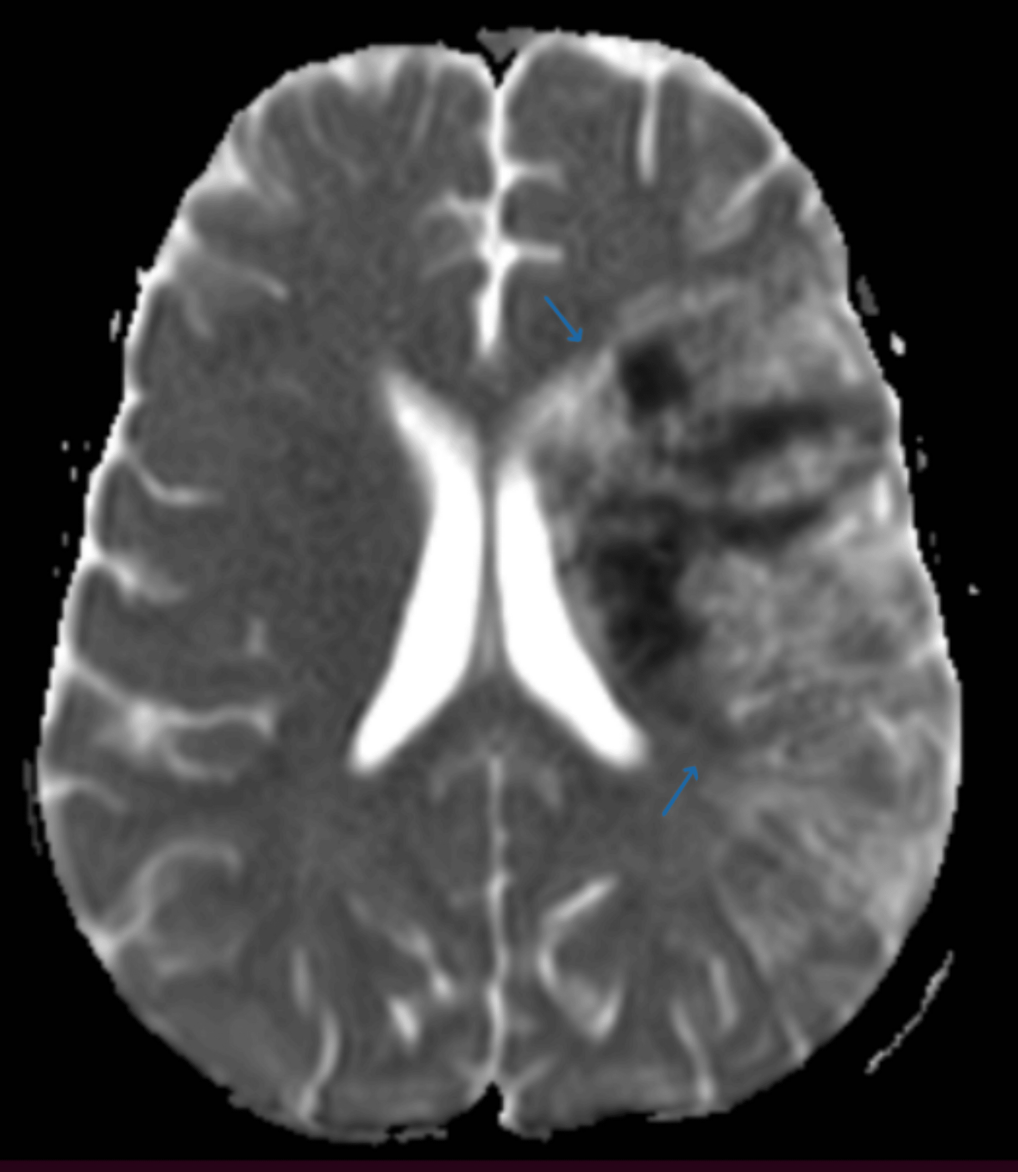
Axial T2-weighted MRI brain showing hyperintense signal in the left frontoparietal cortex and subcortical white matter, consistent with acute infarction involving the left MCA territory. MCA: middle cerebral artery
